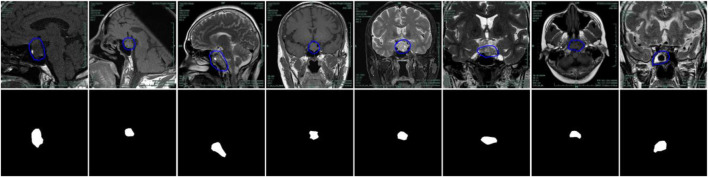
Examples of problems in segmenting pituitary adenoma from MR images. The first row: original images. The second row: the gold standard sketched by experts.
